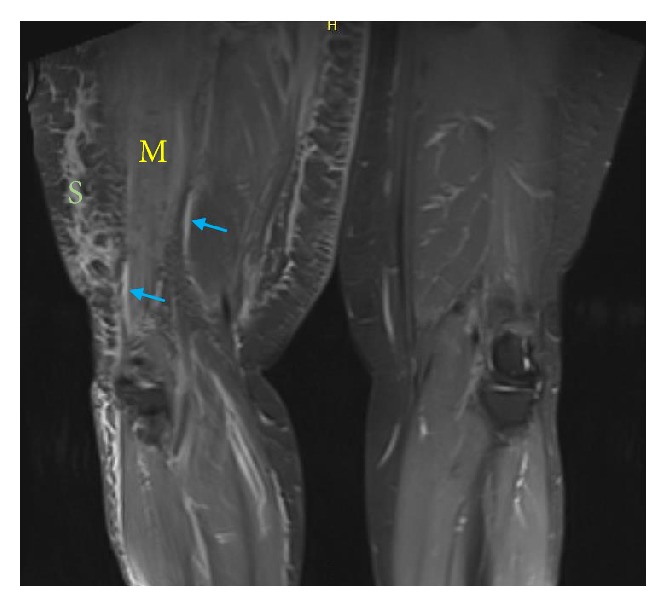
MRI leg without contrast. Right thigh musculature (M) and subcutaneous tissues (S) with diffuse swelling and edema-like signal on T1-weighted imaging. Fluid-like signal is noted at the fascial planes (arrows).
